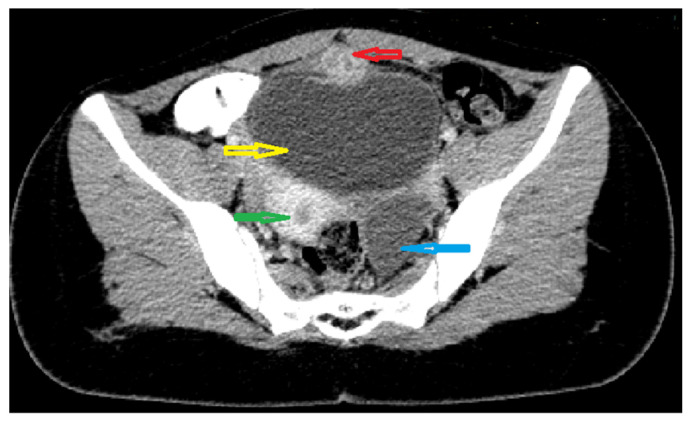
Axial CT scan of abdomen and pelvis with intravenous and oralcontrast: red arrow; supposed urachal cyst; yellow arrow: urinary bladder;green arrow: uterus; blue arrow: left ovarian cyst.
